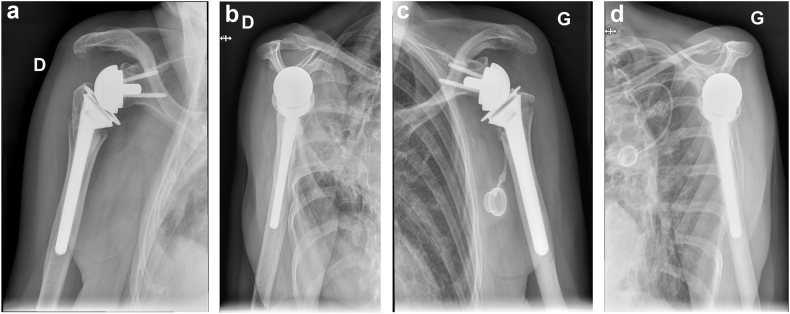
X-ray (anteroposterior, Neer views) 3 months postoperative of Right (a, b) shoulder and Left (c, d) shoulder, showing correct positioning of reverse total shoulder arthroplasty and healed tuberosities on both sides.
